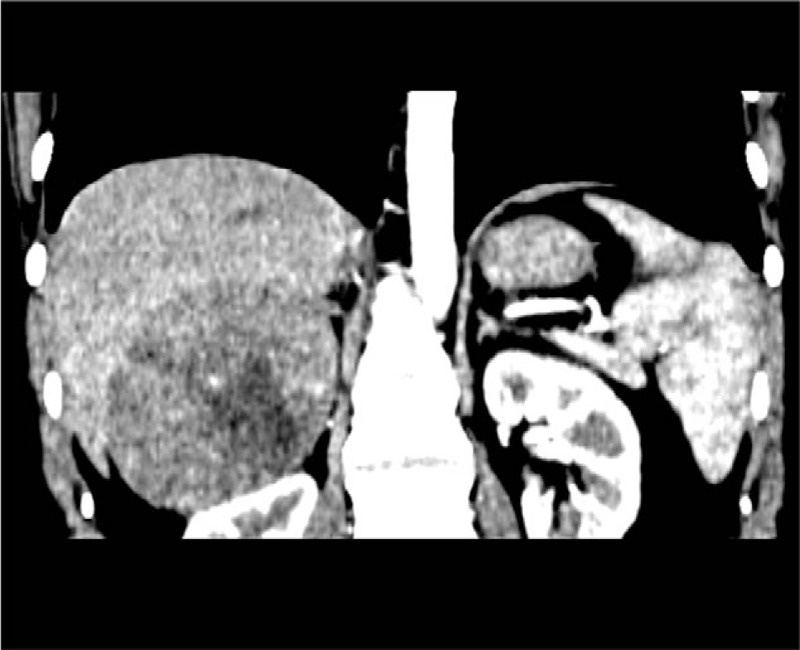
The representative CT scan showed the heterogeneous enhancement of the tumor located between the liver and the right kidney, and necrosis can be seen within the tumor. CT = computed tomography.
